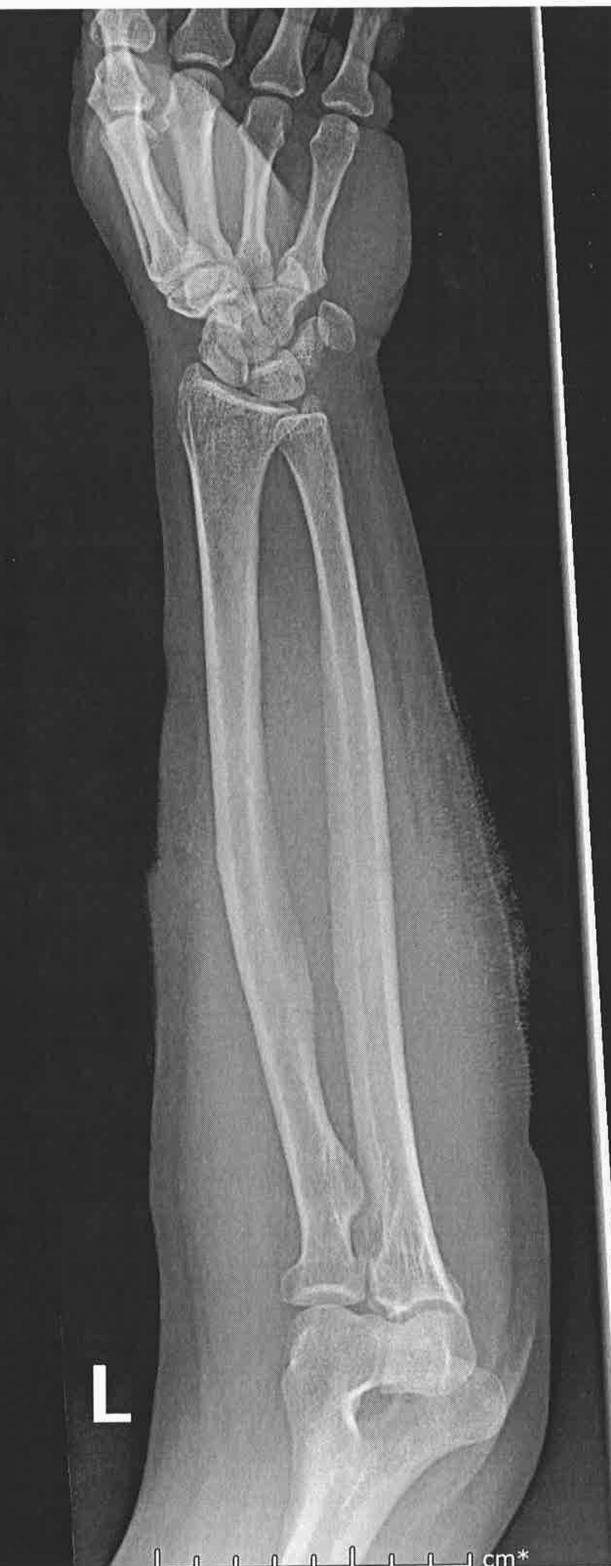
X-ray of left forearm displaying crepitus AP view
